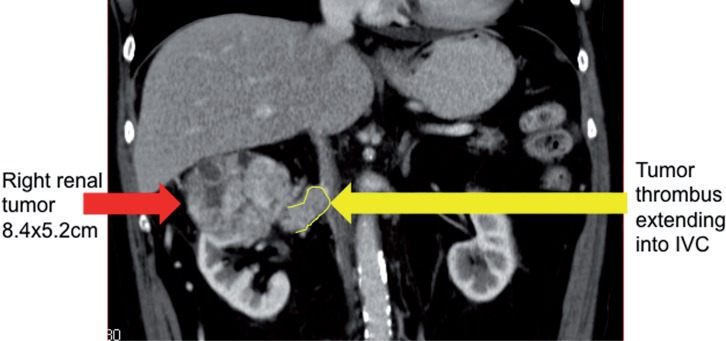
Picture showing pre-operative CT scan showing right renal tumor with thrombus extending into IVC at the level of renal vein.
